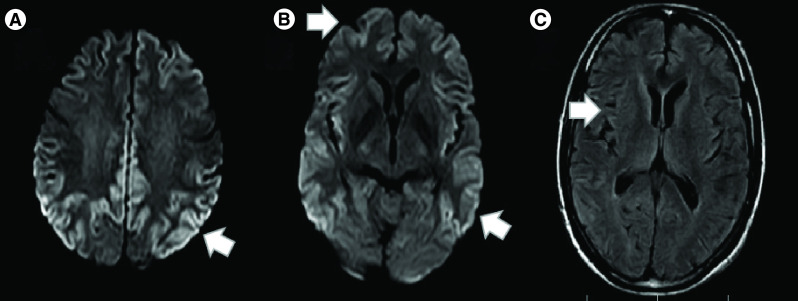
Brain MRI performed on the second day of hospital admission. (A & B) Brain MRI, diffusion-weighted imaging and (C) fluid-attenuated inversion recovery sequences demonstrated global parenchymal loss as well as bilateral high-intensity areas affecting the frontal, parietal, temporal and occipital cortices (cortical ribbon sign).
